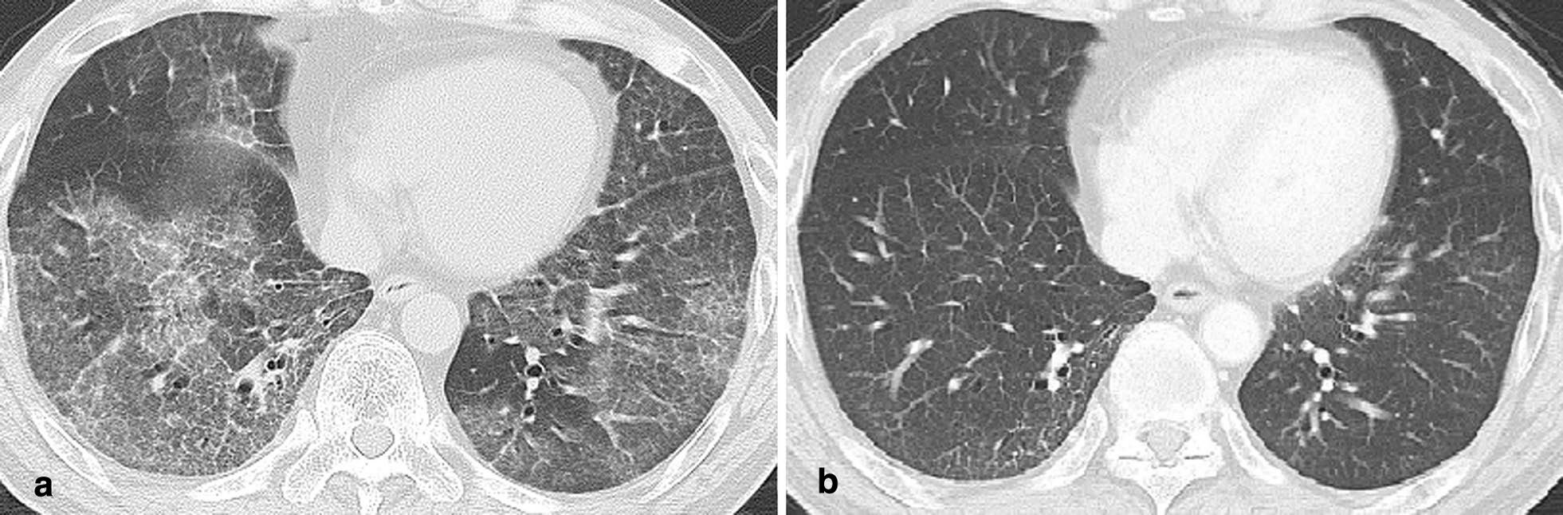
Computed tomography findings of the chest. Ground-glass opacities were seen in the lower lobes of the bilateral lung with a crazy-paving pattern on admission (a). Ground-glass opacities were improved after antibiotic therapy (b)
